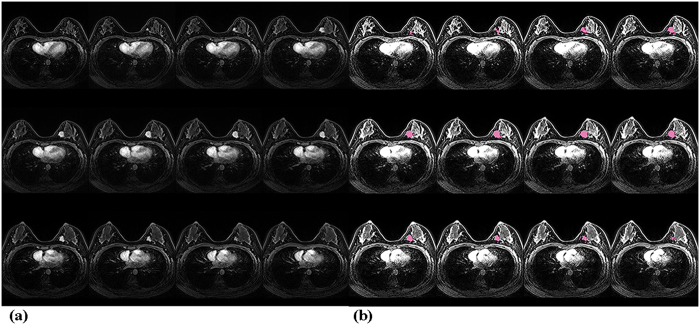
(a) Fat-saturated T1-weighted images with gadolinium enhancement in a patient with breast cancer at the mid-inner portion of the left breast. (b) Applying magic wand tool in post processing program (Olea Sphere, version 3.0) to extract and analyze the enhancing portion of the tumor in the same patient.
